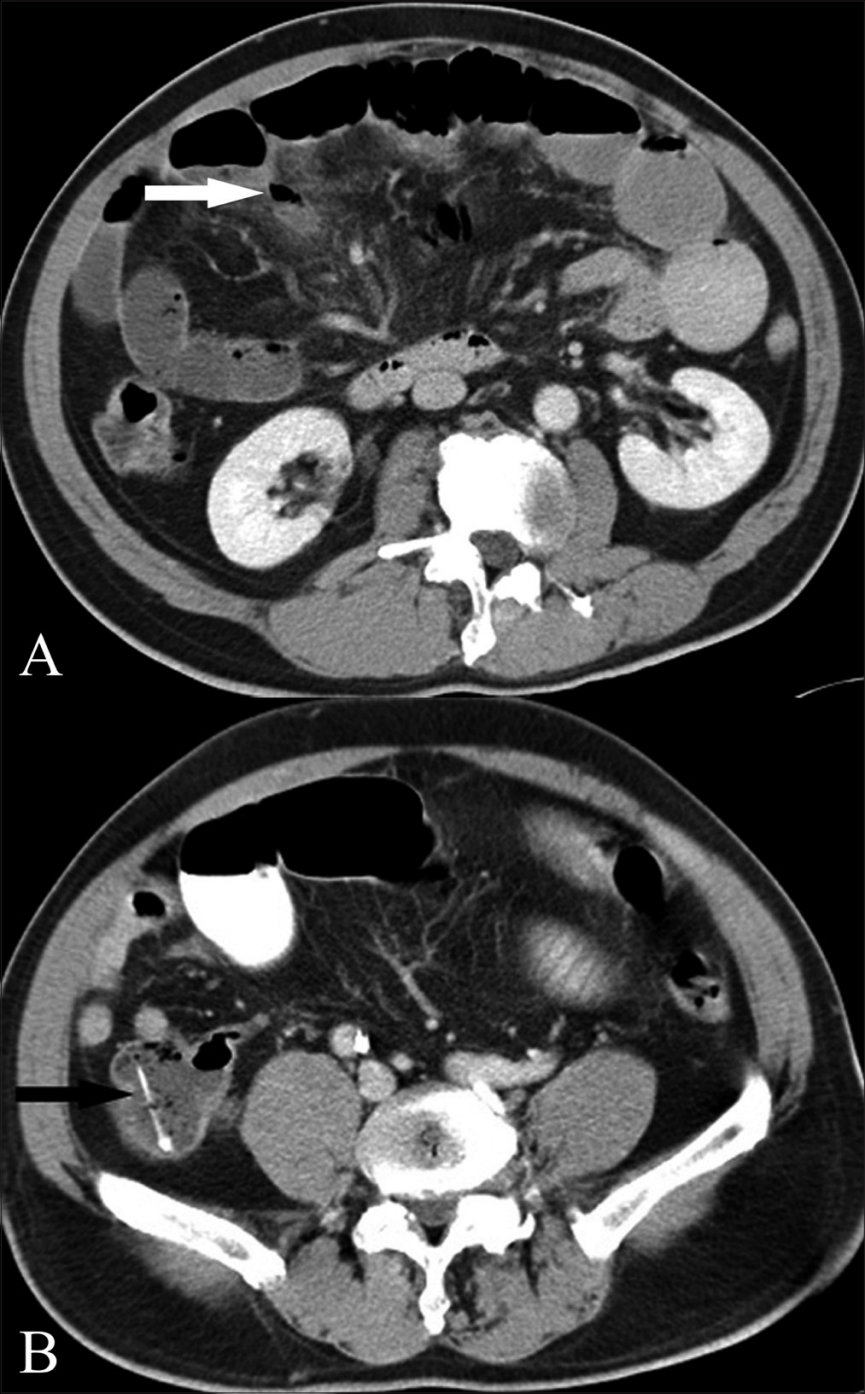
Axial contrast-enhanced CT scans show focal fat stranding and localized pneumoperitoneum in relation to the ileal loops (white arrow in A). There is associated dilatation of the bowel loops, suggesting obstruction. The FB is seen distally in the large bowel (black arrow in B), reemphasizing the importance of closely scrutinizing the bowel loops distal to the inflamed segments
